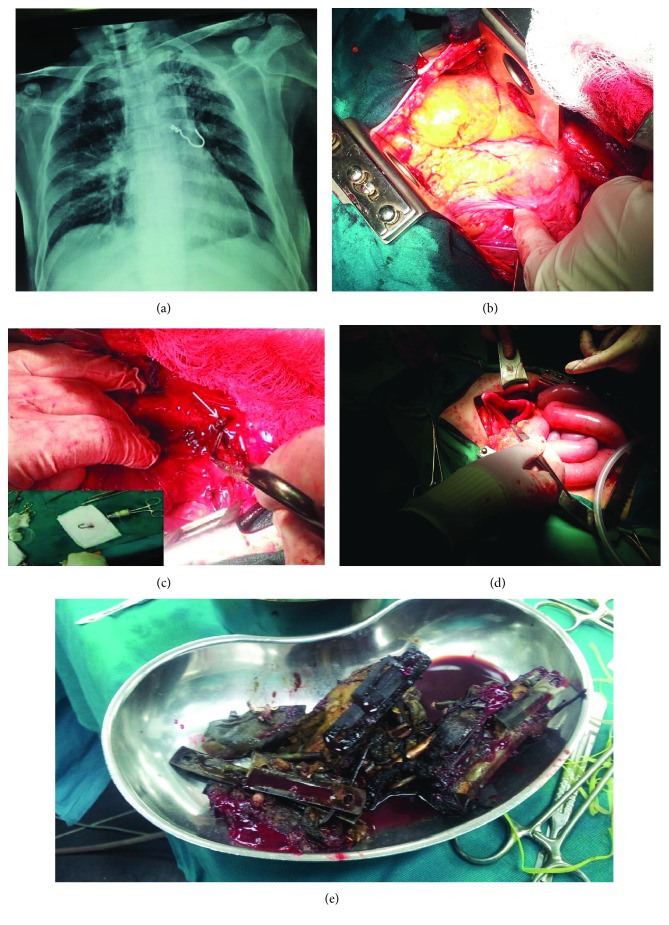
The first episode of FB aspiration and ingestion. (a) PA radiography on admission. (b) Mediastinal emphysema at thoracotomy. (c) FB (arrow) protruding through the left main bronchus (insert: FB removed). (d) Laparotomy and gastrotomy. (e) Metallic FBs removed from the stomach.
